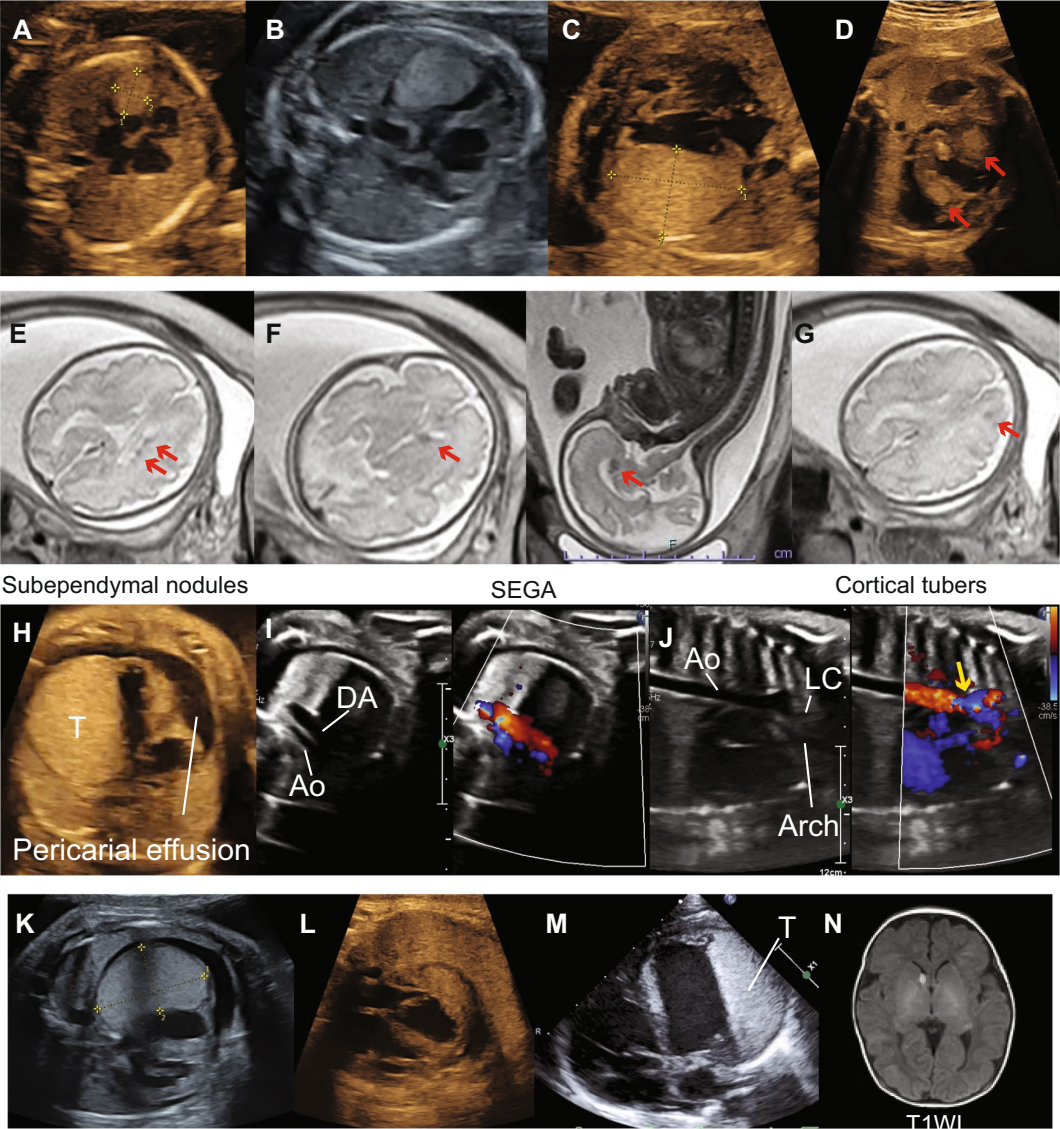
Changes in the size of cardiac rhabdomyomas in the fetus and after birth. A–C The tumor showed a gradual increase in size. The size increased to 15.6 × 12.9, 24.1 × 19.1, and 28.8 × 19.9 mm at A 24, B 26, and C 28 weeks of gestation, respectively. D In addition to the large cardiac tumor, multiple cardiac tumors (red arrows) were found at the septum and appendix. E–G Fetal MRI at 31 weeks of gestation revealed three characteristic cranial tumors: E subependymal nodules (horizontal section), F SEGA (horizontal and sagittal section), and G cortical tubers (horizonal section). The size of SEGA was 7.9 mm in diameter. H Cardiothoracic ratio > 50% with large pericardiac effusion. T indicates tumor lesion. I Decreased LV function similar to that of hypoplastic left heart. Transverse flow in the aorta compared to the flow in the ductus arteriosus. Aberrant flow in the aortic arch. J Blood flow for three common carotid arteries were supplied from the right ventricle through the ductus arteriosus (at 33 weeks of gestation before sirolimus treatment). K Cardiac tumor after two weeks of maternal sirolimus treatment. The tumor size decreased to 37.2 × 22.8 mm at 35 weeks of gestation. L The cardiac tumor was significantly decreased after five weeks of sirolimus treatment. The size of the tumor decreased to 26.2 × 13 mm, without pericardial effusion. The cardiovascular profile score (CVPS) improved from 7 to 9. M Cardiac echocardiography on postnatal day 0. The largest tumor was 36 mm in the LV lateral wall. The LV could bear the systemic circulation. N Neonatal MRI at postnatal day 14 showing SEGA. The size of the SEGA was 6.1 mm in diameter, which was smaller than that in the fetal MRI at 31 weeks of gestation (7.9 mm in diameter). MRI, magnetic resonance imaging; SEGA, subependymal giant cell astrocytoma; LV, left ventricle; T, rhabdomyoma tumor; DA, ductus arteriosus; Ao, aorta; LC, left common carotid artery
